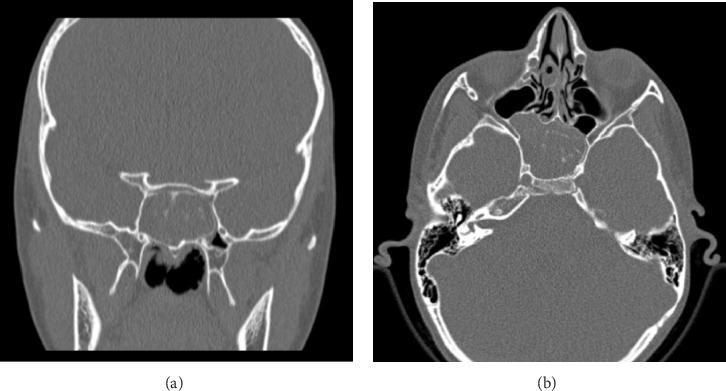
Brain CT showing complete opacification of the sphenoid sinus with erosion of the sphenoid septum and multiple hyperdense deposits: coronal view (a); axial view (b).
